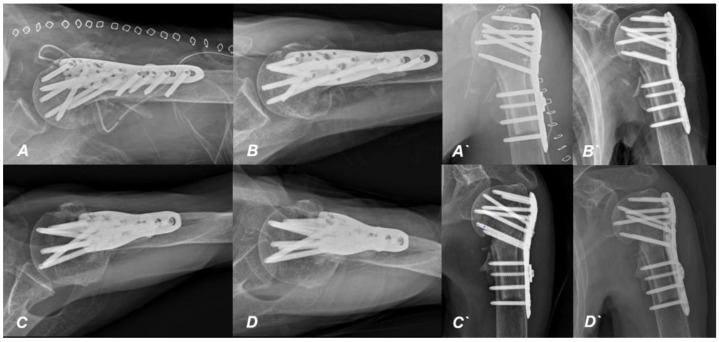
Serial plain radiographic images during the postoperative follow-up period. Continuous radiographic reviews were conducted throughout the outpatient follow-up period following surgery. The images depict radiographs taken at immediate (A,A′), 3 months (B,B′), 6 months (C,C′), and 1 year (D,D′) postoperatively.
